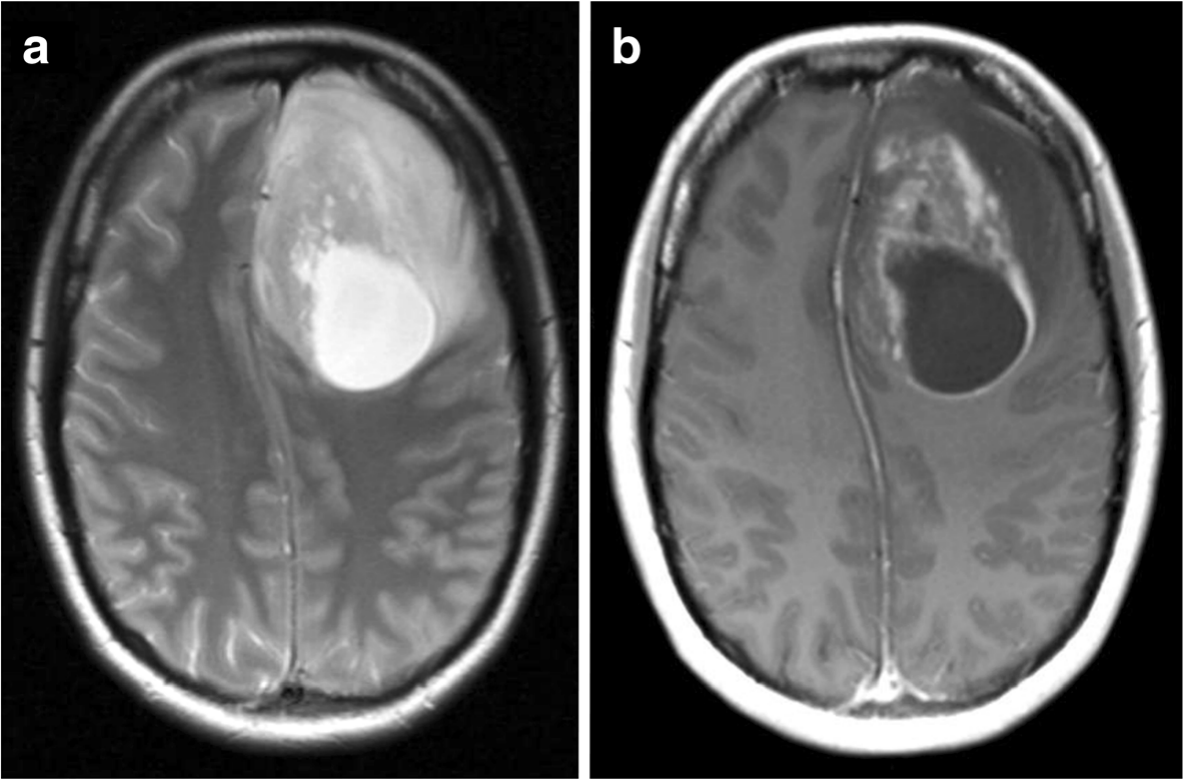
A heterogeneous tumor (65 × 50 mm) in the left frontal lobe with solid and cystic segments partly showing strong, irregular, and ring-shaped contrast enhancement. a T2-weighted image. b T1-weighted image with contrast agent
